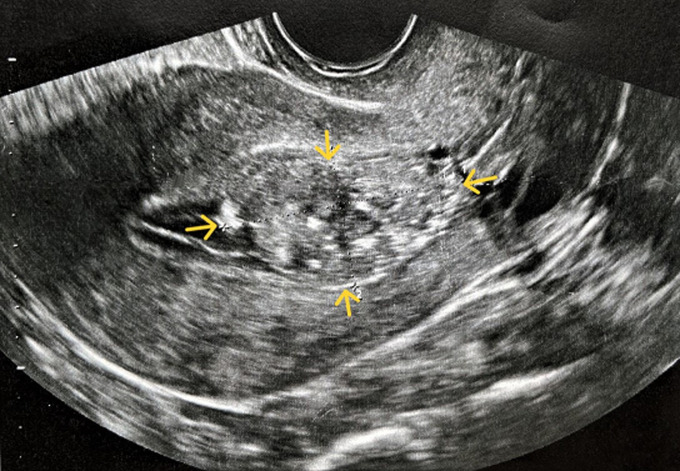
transvaginal ultrasound imaging of an atypical polypoid adenomyoma of the uterus; a solid, round-shaped, well-defined mass (yellow arrows) within the endometrial cavity is easily visualized
